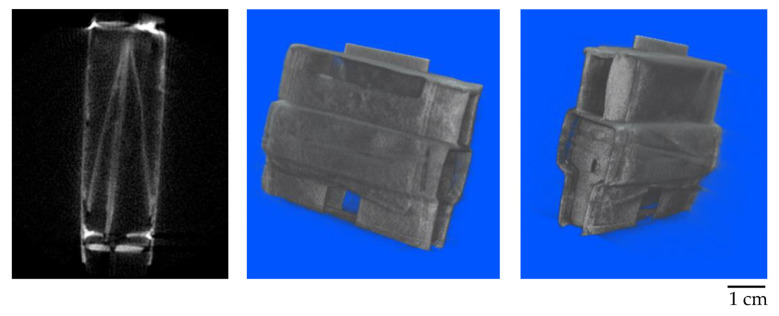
Results of first neutron computed tomography at 500 W, a tomographic slice and 3D views.
